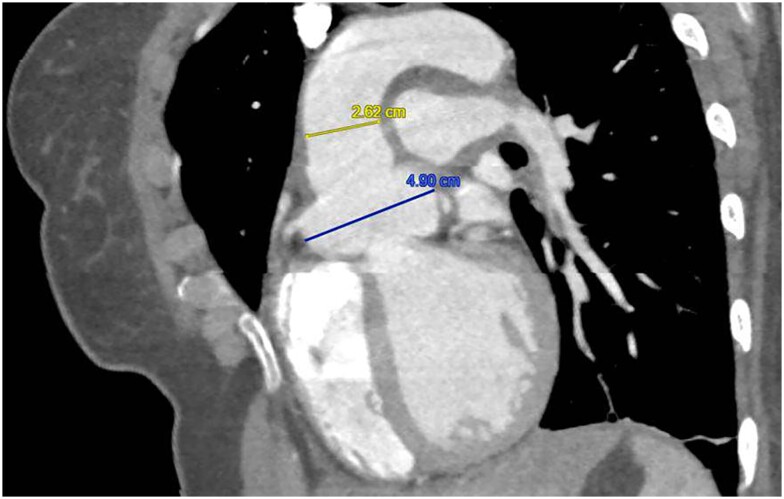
Computed tomography aorta in sagittal view demonstrating aneurysmal dilatation of the right and left sinuses of Valsalva with aortic root diameter of 4.9 cm. The proximal ascending aorta measured normal at 2.62 cm; 94 × 149 mm.
